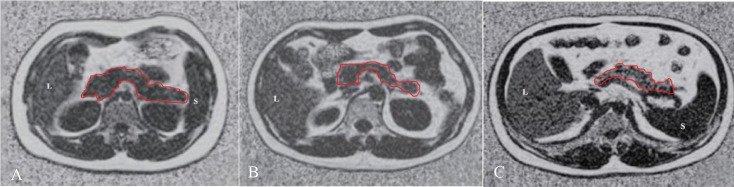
Representative quantitative pancreatic fat fraction mappings (Dixon MRI) across the glycemic continuum. Red contours delineate regions of interest (ROIs) manually segmented along anatomical parenchymal boundaries, strictly excluding peripancreatic adipose tissue. (A) Normoglycemic control (NGT) demonstrating uniform, healthy parenchyma. (B) Impaired glucose tolerance (IGT) showing intermediate lipid accumulation. (C) Newly diagnosed T2DM exhibiting severe, diffuse fatty infiltration. L, liver; S, spleen. Adapted and modified from Liang et al. (36), originally published in Journal of Sun Yat-sen University (Medical Sciences) under the terms of the Creative Commons Attribution-NonCommercial 4.0 International License (CC BY-NC 4.0).
